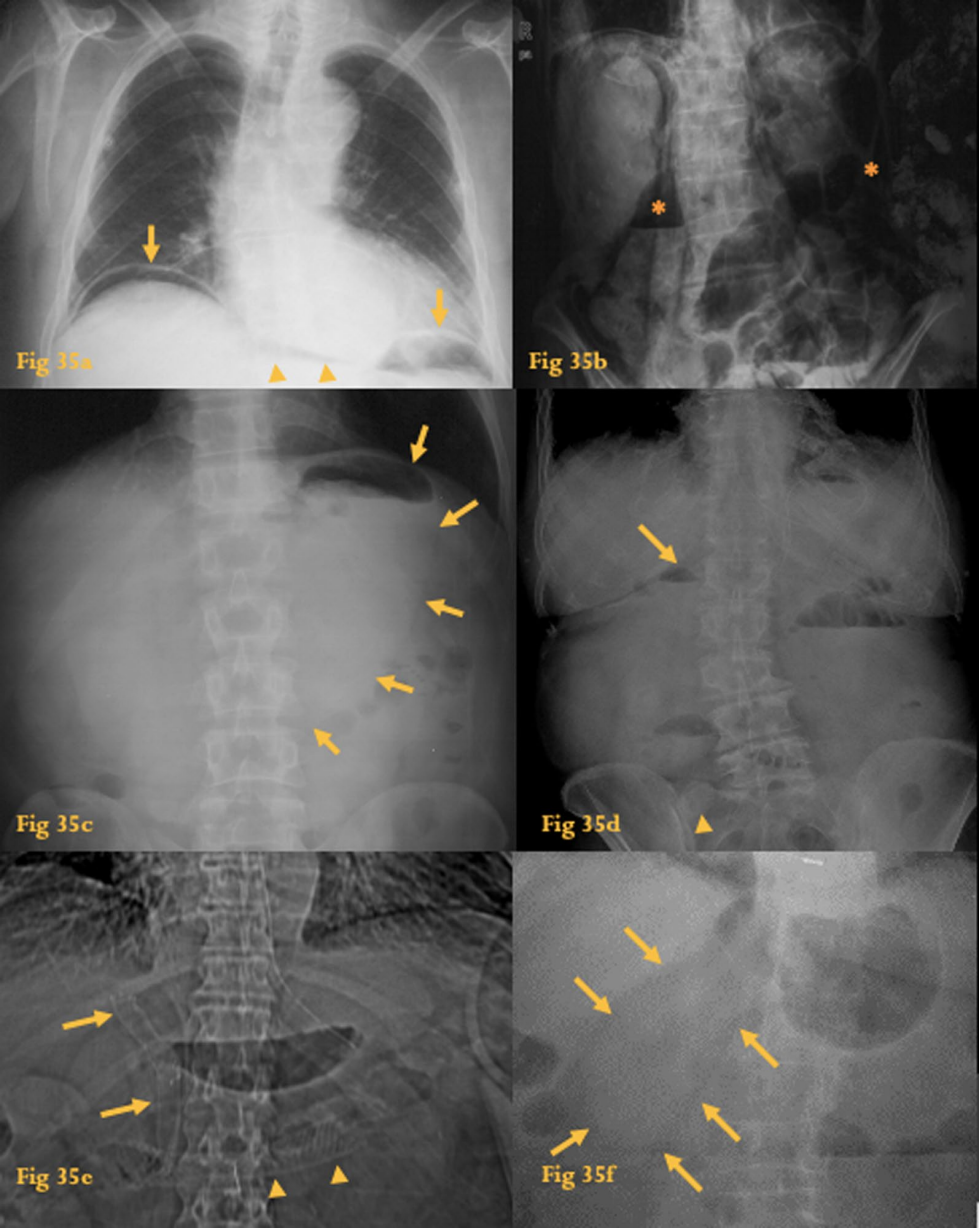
Indirect signs of duodenal disease on X-ray. Chest radiograph (a) shows a small amount of gas beneath the diaphragm (arrows) and the continuous diaphragm sign is also seen (arrowheads). At surgery, a perforated duodenal bulb ulcer was identified. Abdominal radiograph (b) shows pneumoretroperitoneum (asterisks) after duodenal rupture following ERCP. Abdominal radiograph (c) shows a stomach with augmented volume (arrowheads)—gastric outflow obstruction caused by multiple polyps of the duodenum in a patient with juvenile polyposis. Abdominal radiograph (d) shows an air-fluid level in the gallbladder (arrow) and a gallstone at the right iliac fossa (arrowhead), indicative of a gallstone ileus, because of a cholecystoduodenal fistula. Abdominal radiograph (e) shows a large horizontal prosthesis in the upper abdomen (arrowheads) and two smaller vertical prosthesis (arrows) in the horizontal and descending duodenum, which were a palliative treatment for an obstructive duodenal adenocarcinoma. Abdominal radiograph (f) shows an intraluminal soft-tissue density at D2. This was a gastric GIST prolapsing into the duodenum
